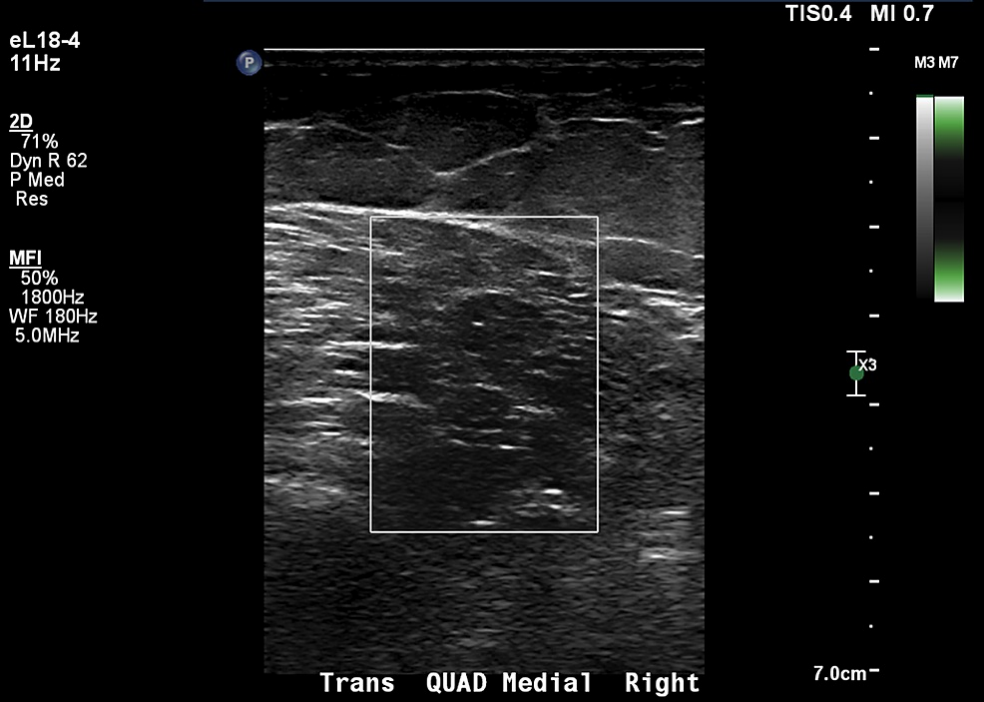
Gray-scale power Doppler ultrasound: transaxial view of the quadriceps muscle (square) showing absent Doppler flow in the context of active myositis flare
